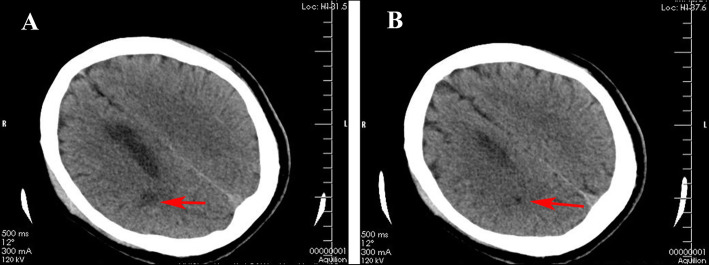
Cranial CT shows cerebral infarction anterior to the anterior horn of the right ventricle (red arrow)
